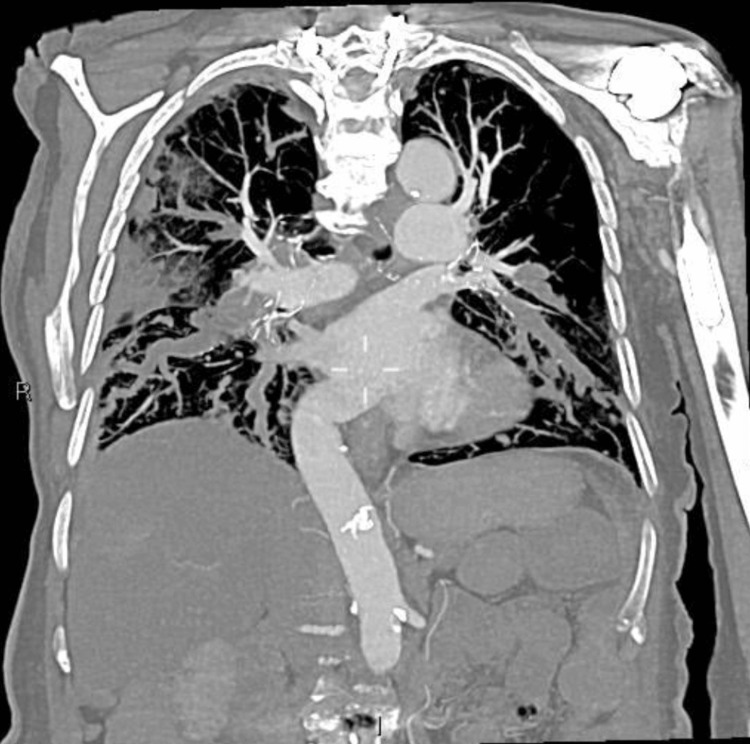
Coronal CT of the chest from staging chest-abdomino-pelvic CT six months after the initial presentation. The image demonstrates progressive bilateral tumour thrombi in pulmonary arterial branches with luminal distension.
